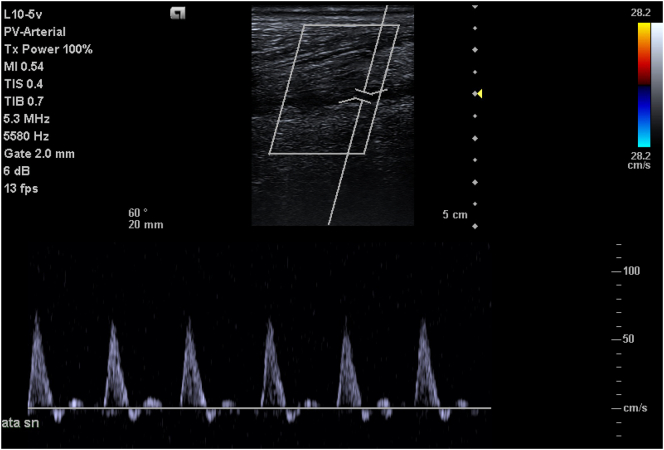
Ultrasound check of anterior tibial artery.
